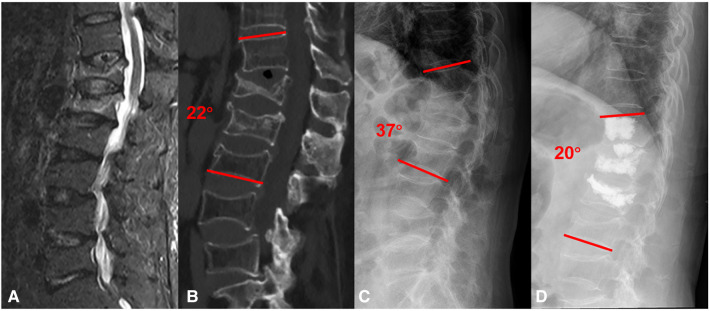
A 76-years old female with vsOVCFs and kyphosis at T12-L1 was treated with PVDP. (A) Magnetic resonance imaging (MRI) at admission showed T12-L1 severe compression fracture with kyphosis in preoperative. (B) Supine CT scan at admission showed the local kyphosis angle was 22° and T12-L1 with vacuum phenomenon. (C) The lateral standing x-ray showed the local kyphosis angle was 37°, the different angulation due to the vertebral and intervertebral collapse (22° and 37°) called the accordion phenomenon. (D) Postoperative 12-month x-ray showed that cement was injected in the vertebra and the middle of the T12-L1 intervertebral space and kyphosis was corrected, the local kyphosis angle (20°) was maintained.
